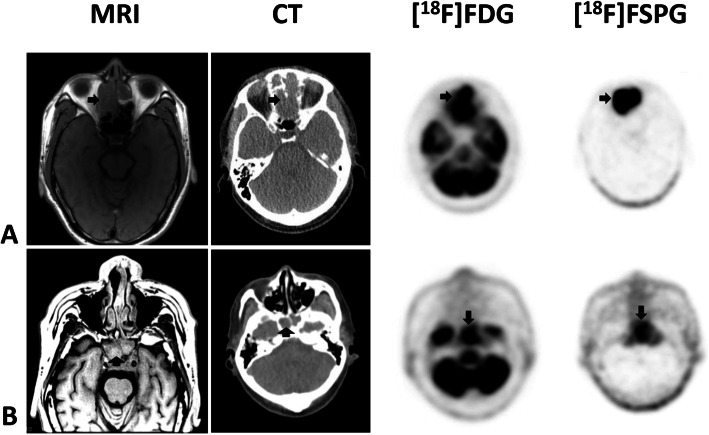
Combined image set shows two representative subjects with primary head and neck cancer (HNC). Shown from left to right are the axial T1-weighted MRI, CT, [^18^F]FDG PET, and [^18^F]FSPG PET images. The first subject (row a) has a large nasal mass while the second subject (row b) has a mass in the clivus/nasopharynx. The black arrows indicate the lesions. Any unmarked uptake on the PET scans is physiologic. All modalities show the lesions, although MRI and PET are clearer than CT. The relative uptake of [^18^F]FSPG and [^18^F]FDG within all 5 HNC subjects is similar (SUV of 2.76 ± 2.13 for [^18^F]FSPG and 4.92 ± 2.16 for [^18^F]FDG), although the complete lack of [^18^F]FSPG background uptake in normal brain (SUV of 0.1) versus [^18^F]FDG (SUV of 7.88 ± 1.45) makes evaluation of the [^18^F]FSPG images much easier and more suitable, for example, in radiation treatment planning
